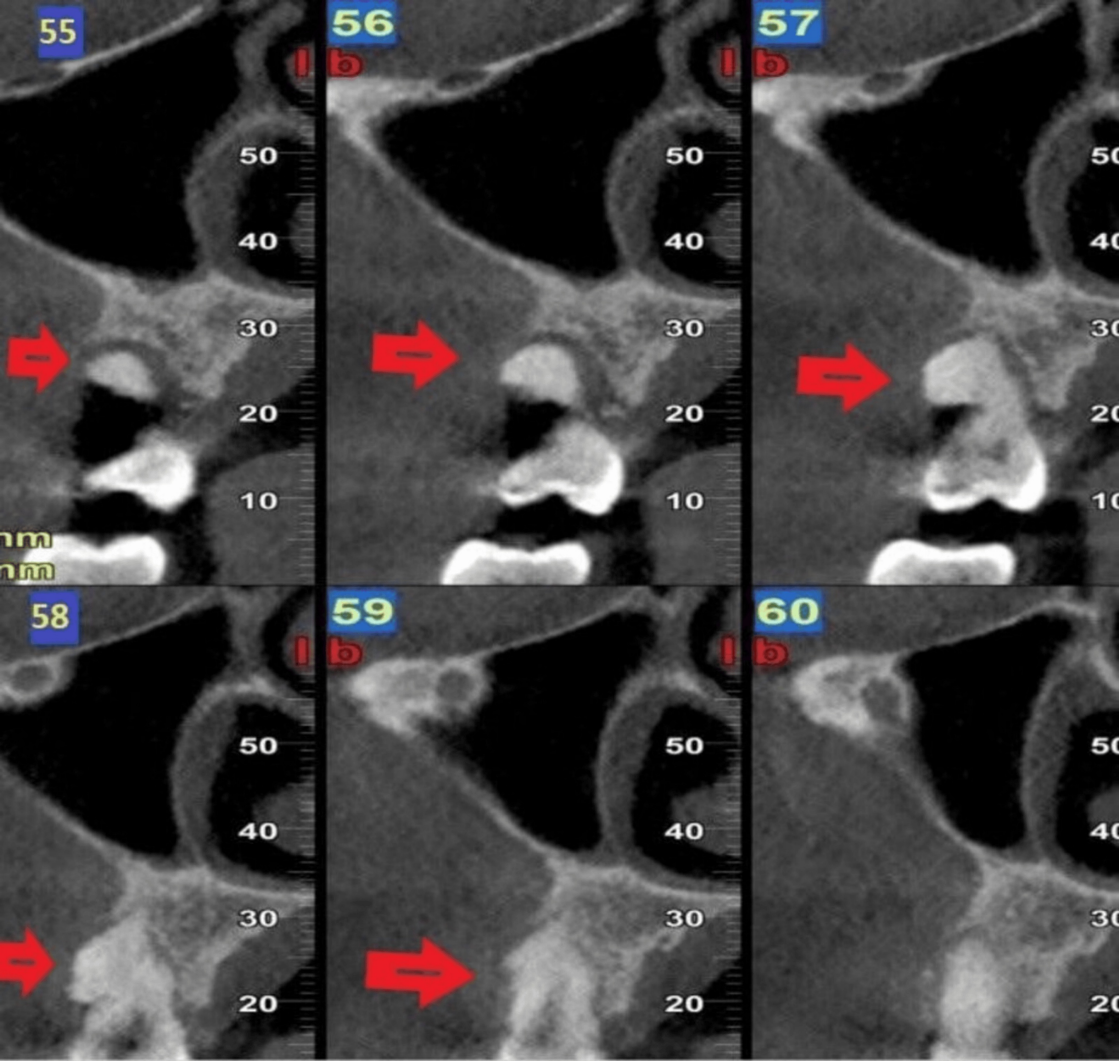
Images 55, 56, 57, 58, and 59 of CBCT paraxial cuts from distal to medial showing the lesion Images 55, 56, 57, 58, and 59 of CBCT paraxial cuts from distal to medial showing the lesion diameter and attachment to the second premolar on the upper right jaw (red arrows). The capsule of the tumor is also visible. The tumor was not visible on image 60. Here, “l” indicates lingual, and “b” represents buccal, which help the clinician to determine anatomical and pathoanatomical structures CBCT: cone-beam computed tomography
